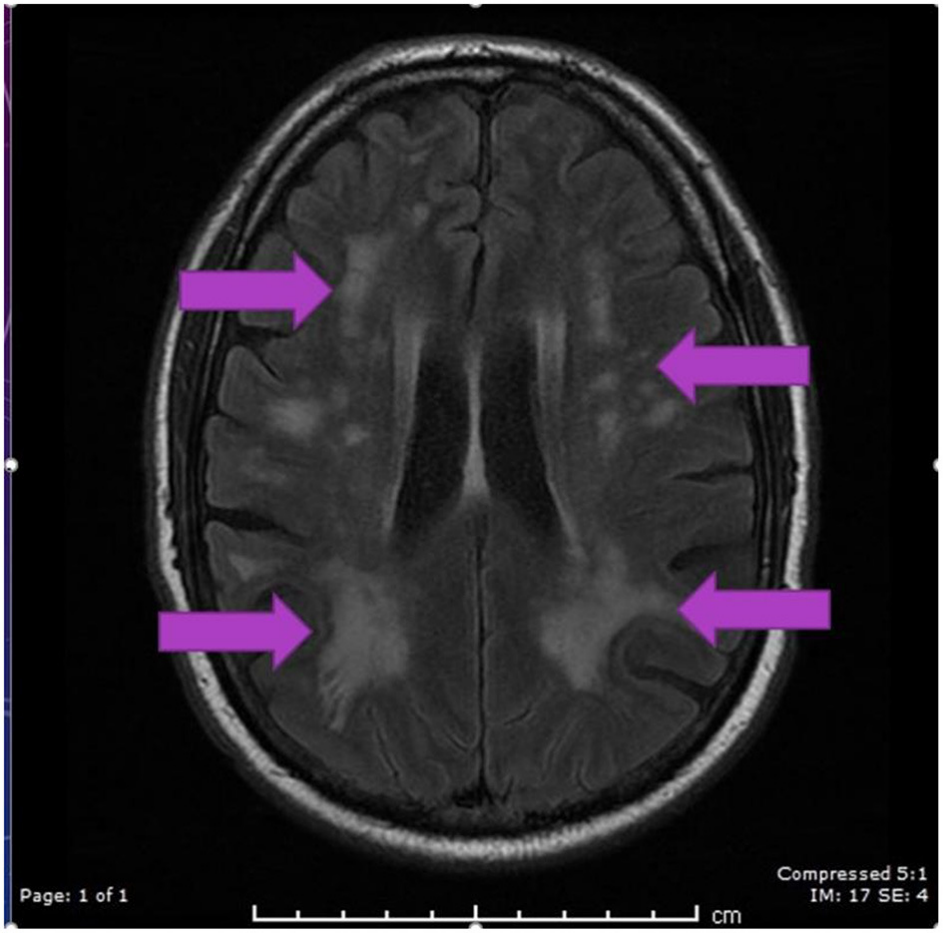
MRI axial FLAIR image demonstrating hyperintensities in periventricular regions in both parietal, occipital and frontal regions secondary to PRES.
